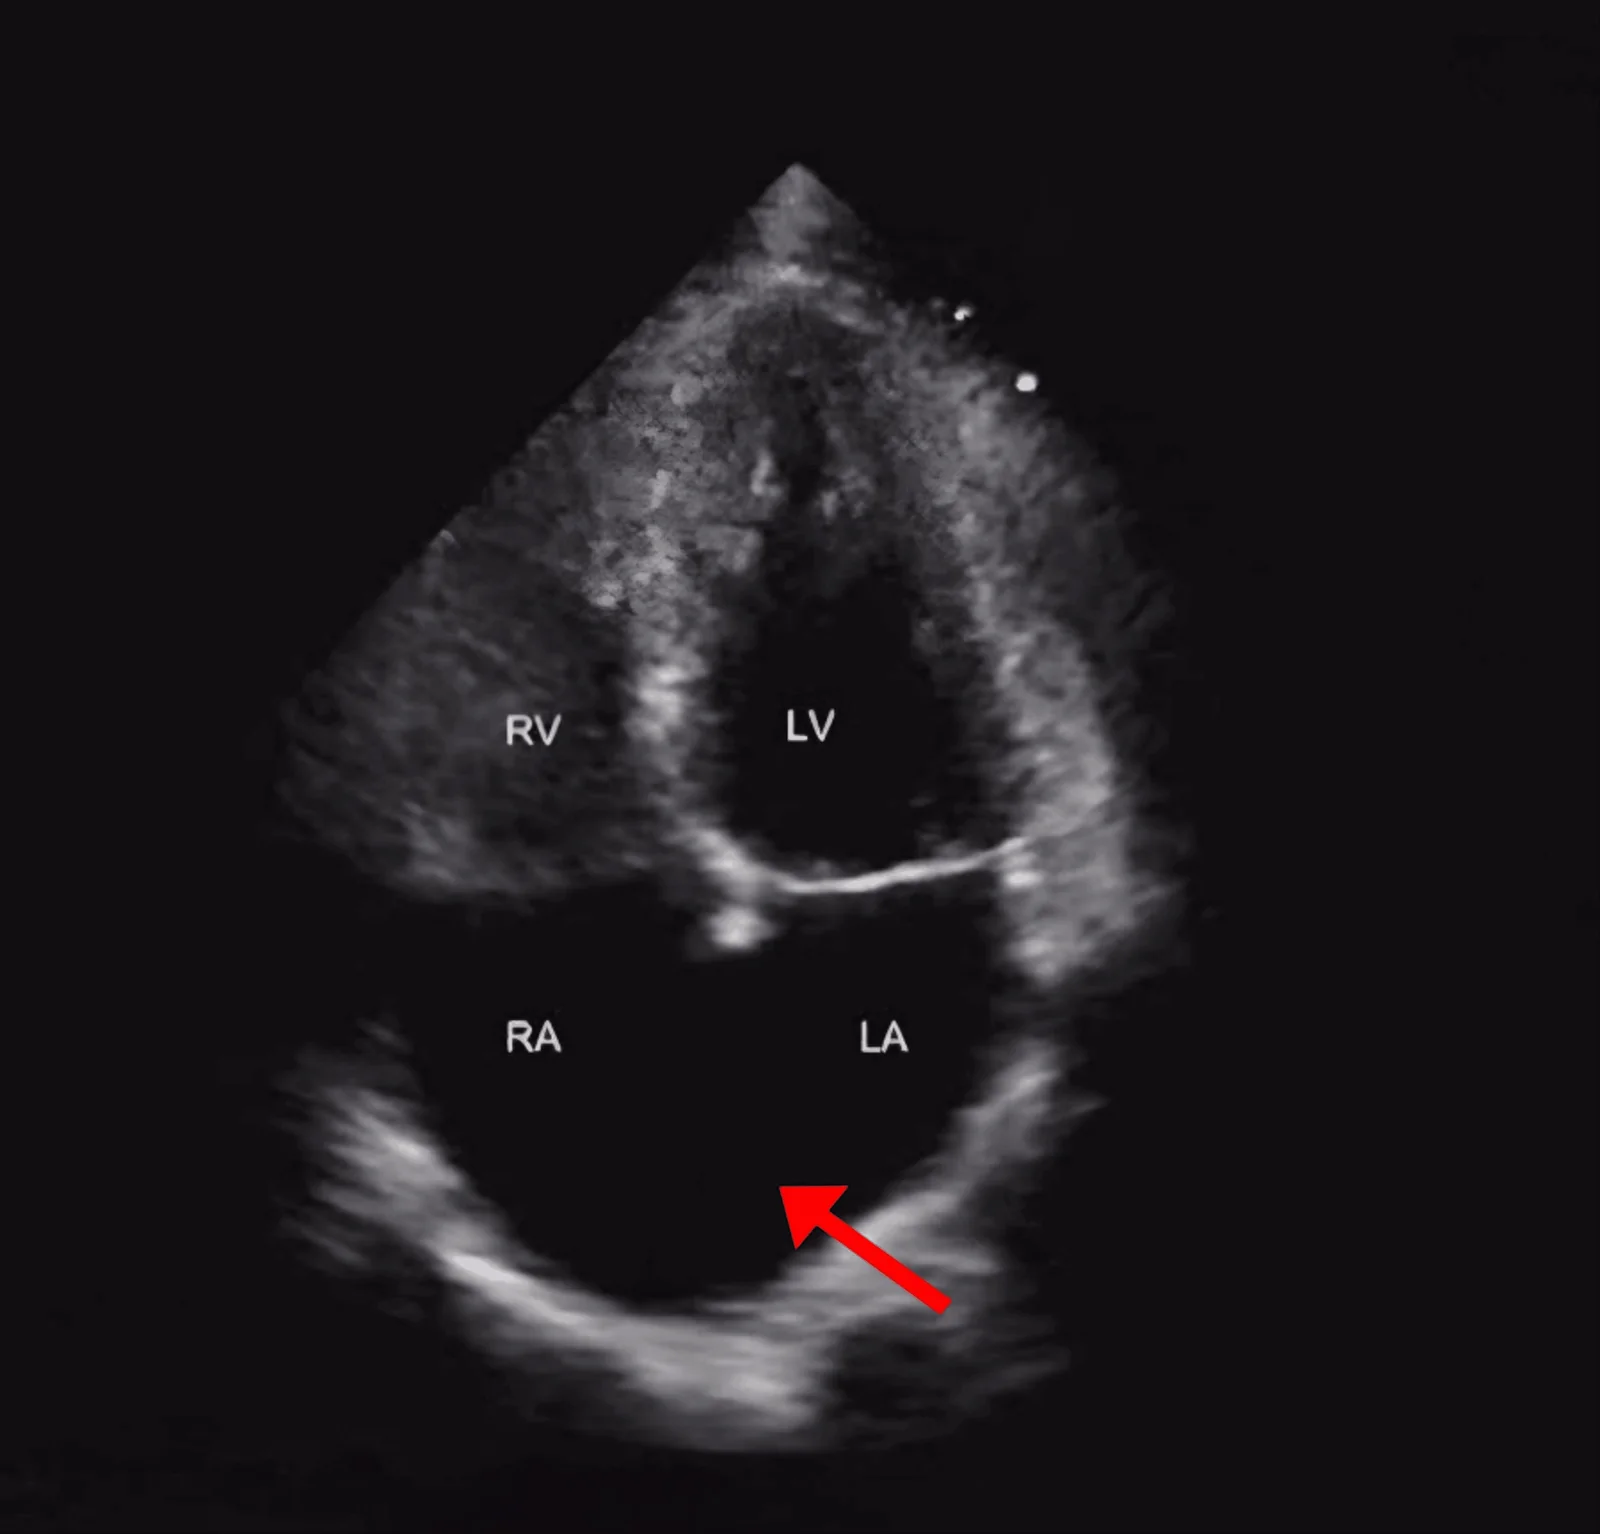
Echocardiography: ostium secundum ASD. Two-dimensional echocardiography apical four-chamber view (RV, LV, RA, and LA): 1.3 cm ostium secundum ASD with color Doppler left-to-right shunt. The red arrow indicates the site of the ostium secundum ASD. ASD = atrial septal defect; RV = right ventricle; LV = left ventricle; RA = right atrium; LA = left atrium
